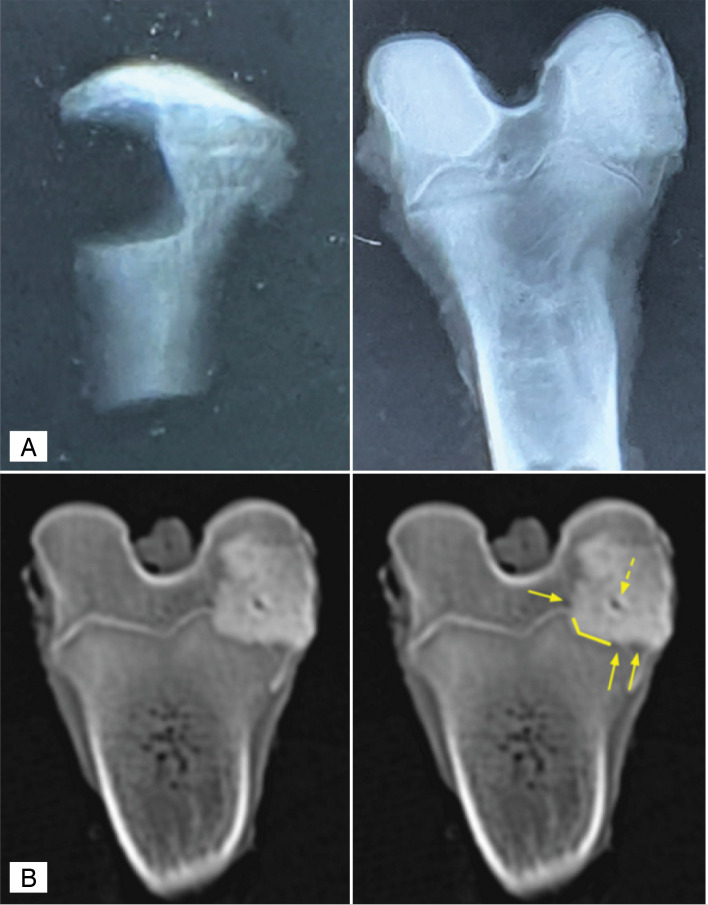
Bone defects in X-ray and computed tomography.
